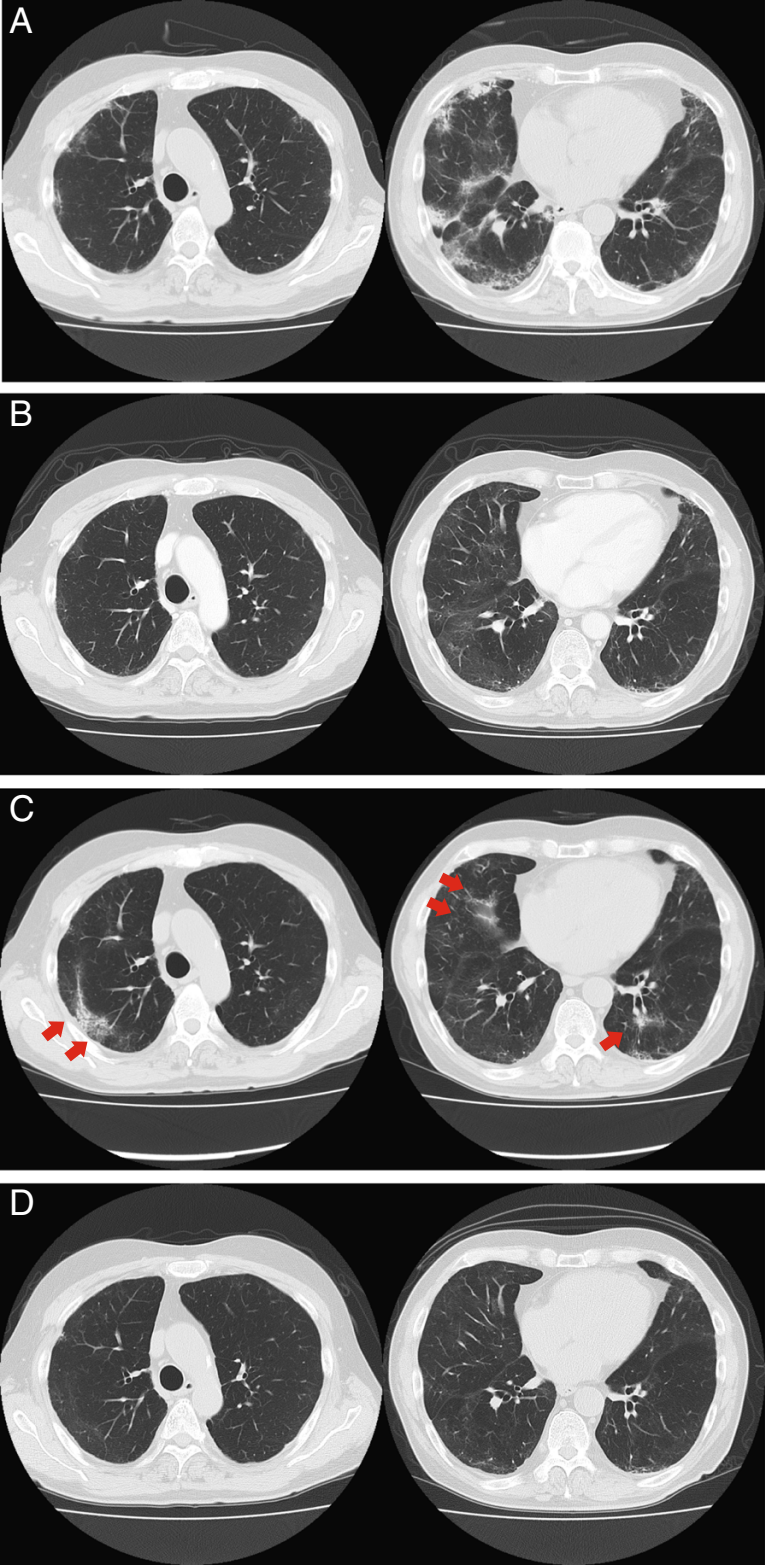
Chest high-resolution CT findings. a Ground glass and dense consolidations with patchy distribution were observed in the bilateral lungs before steroid pulse therapy. b 3 months after steroid pulse therapy, the bilateral dense consolidation seen in (a) was resolved. c 8 months after steroid pulse therapy, newly developed bilateral opacities were seen (arrowheads). d 1 year after the initiation of pirfenidone add-on therapy
